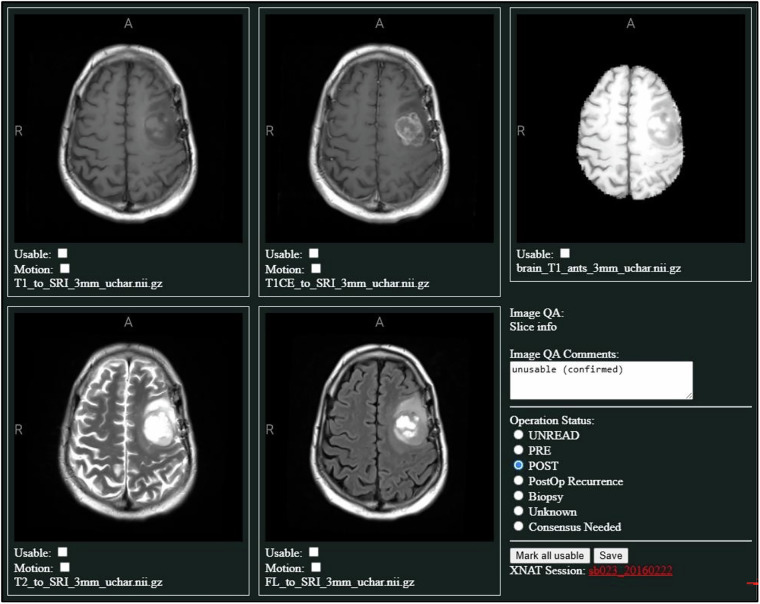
Internal XNAT Tool for MRI Quality Assessment. This figure shows an internal XNAT QA tool to screen preoperative MRI scans. The displayed case was flagged during review due to a left-sided craniotomy. Upon neuroradiologist confirmation, the case was excluded from the final dataset. QA flags like this help ensure the dataset includes only untreated, preoperative images suitable for downstream analysis. The case clearly demonstrates the surgical defect, underscoring the importance of visual inspection during data curation.
